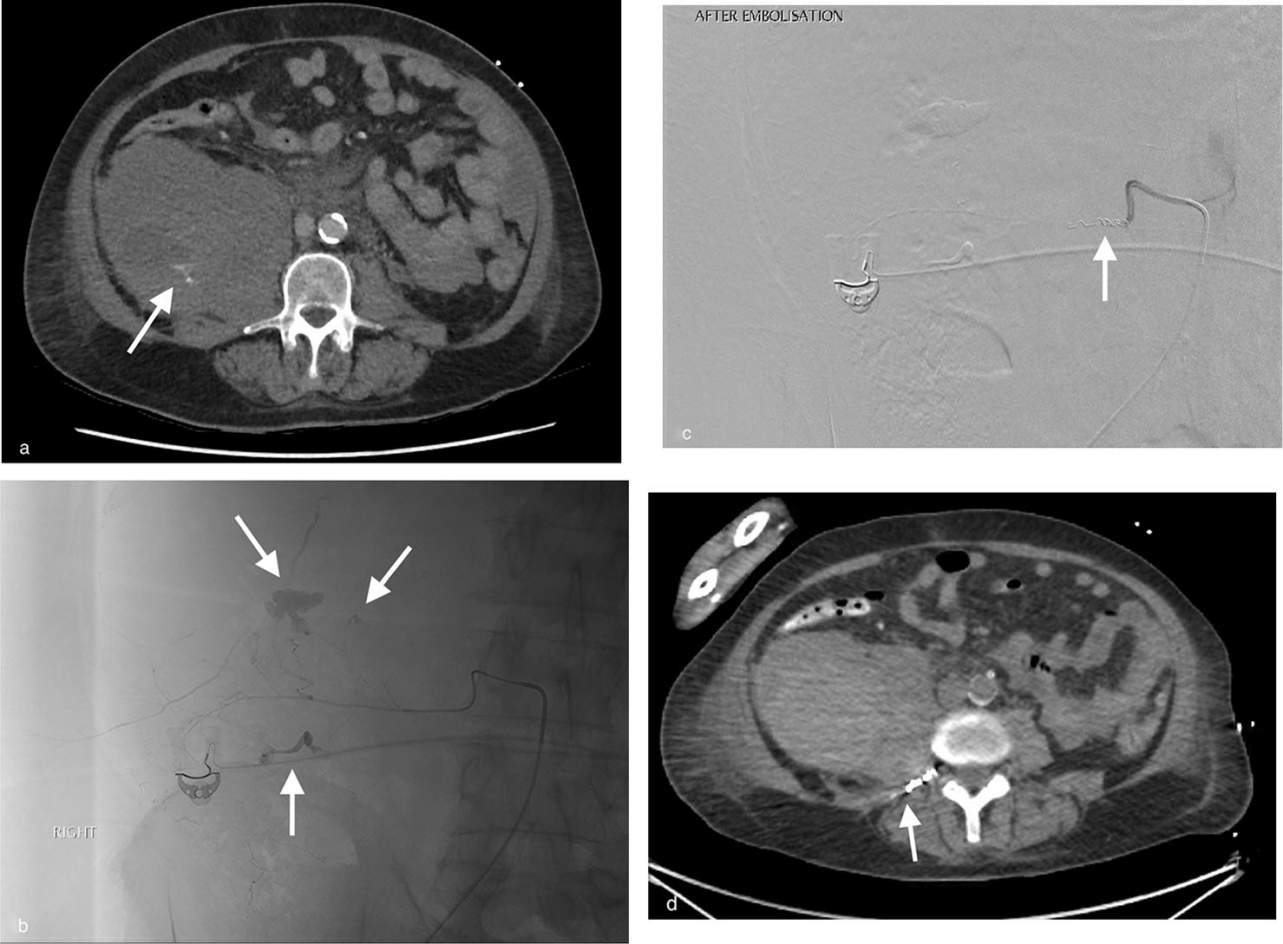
CT of the abdomen and pelvis in delayed contrast phase in a patient on therapeutic heparin showing a large right retroperitoneal haematoma with focus of contrast pooling/blush at the L3 level (a; white arrow); right L3 lumbar artery angiography with contrast injection via a 2.6-Fr microcatheter through a 5-Fr C2 parent catheter showing three foci of active extravasation (b; white arrows); angiography post embolisation with gelatin sponge and 2-mm coils showing stasis of flow (c; white arrow); CT of the abdomen without contrast three days later showing the coils in situ and stable haematoma size (d; white arrow)
